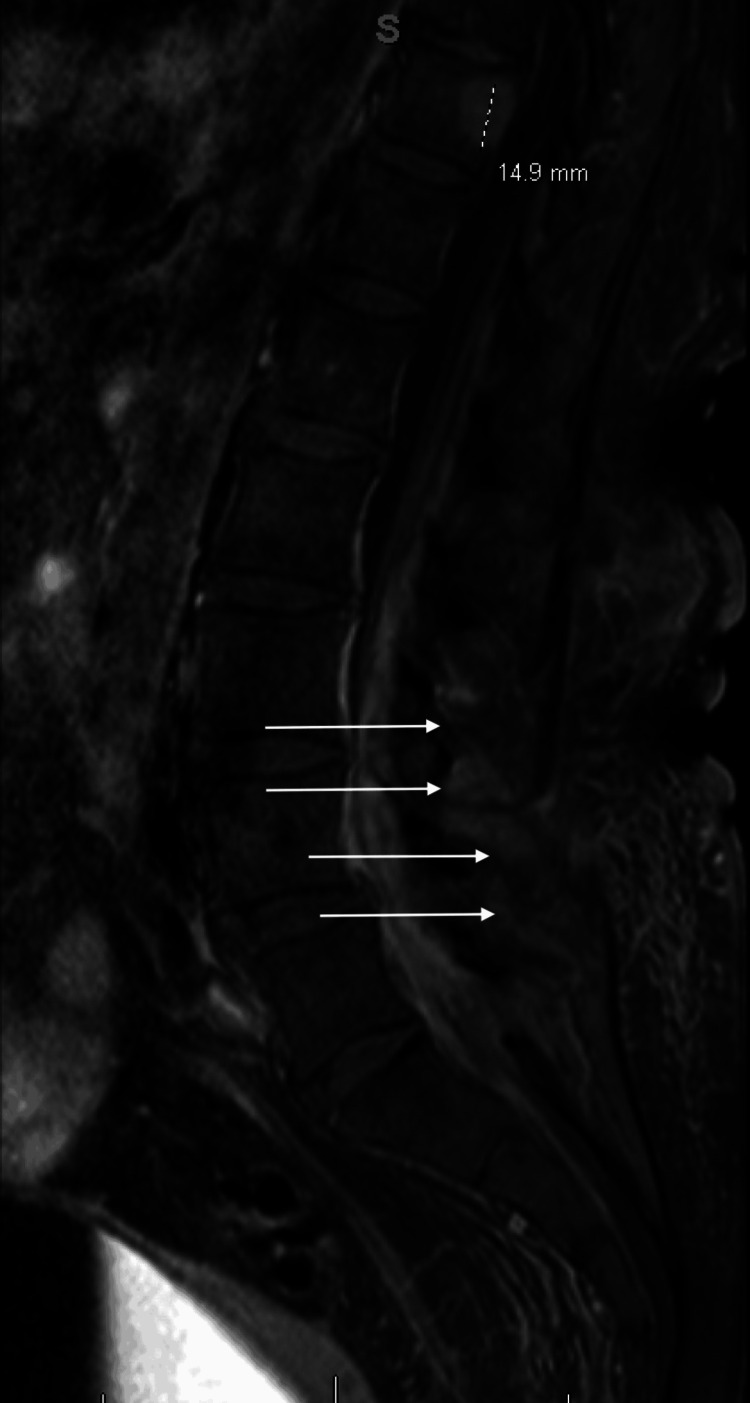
T1-weighted sagittal MRI of the lumbar spine with contrast Key neurological exam findings: 3/5 strength and +1 patellar reflexes in bilateral lower extremities; no saddle anesthesia, Hoffmann sign, dysdiadochokinesia, or clonus. 14.9 mm: At the T11 level, there is enhancement of a benign vertebral hemangioma measuring 14.9 mm in the cranio-caudad dimension, which is far removed from the dural thickening. White arrows: There is enhancement of the posterior paraspinous soft tissues, musculature, and subcutaneous tissues, consistent with myositis and cellulitis.
